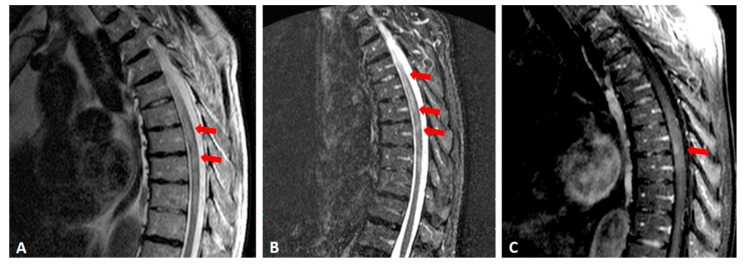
Magnetic resonance imaging showing a longitudinal hyperintense T2 lesion indicated by red arrows in the spinal cord extending from T2 to T11 (A,B) with gadolinium enhancement on T1 sequence at T7–T8 level (C).
